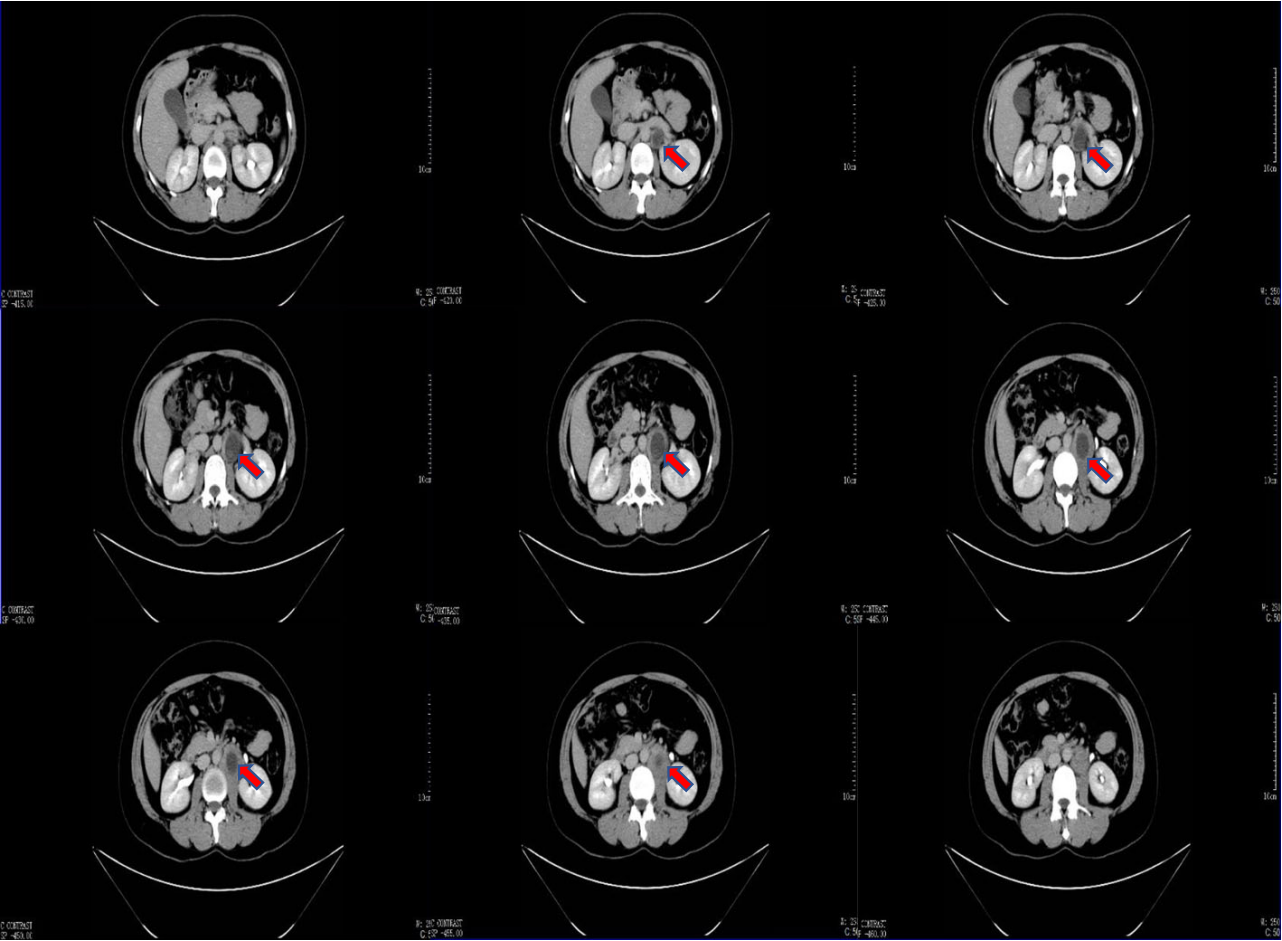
Abdominal computed tomography of Axial plane. The cystic mass was indicated by red arrow was gestational sac
